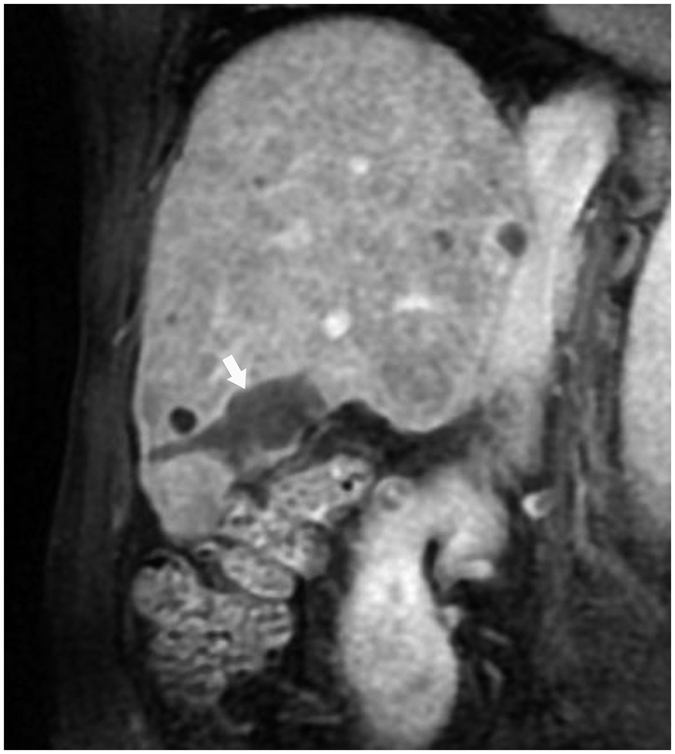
Lesion (white arrow) near the gastrointestinal tract after ablation as shown with enhanced MRI in the coronal view.
